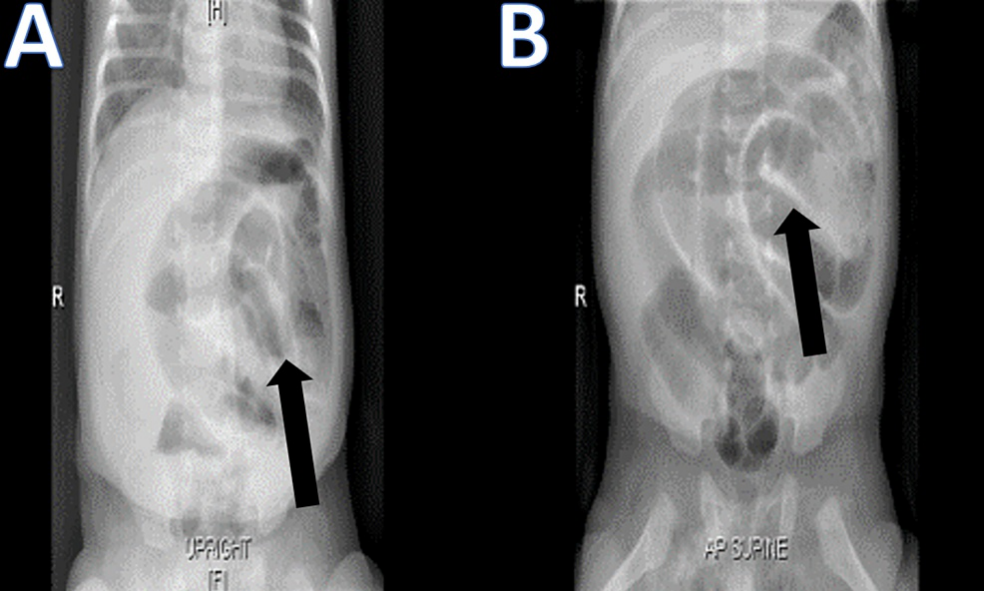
X-ray of the abdomen A: Erect X-ray of the abdomen showed thickened, dilated bowel loops (arrow) with no air-fluid levels. B: Supine X-ray of the abdomen revealed thickened, dilated bowel loops (arrow) with no air-fluid levels.
